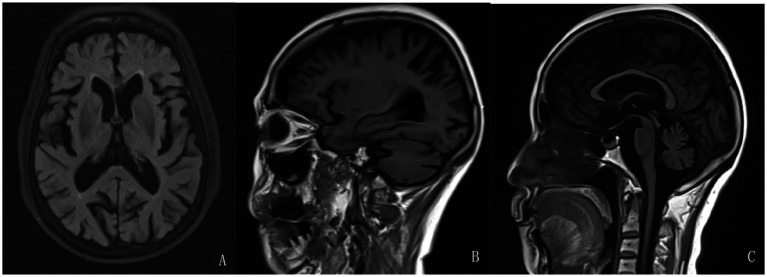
Brain magnetic resonance imaging (MRI) of the patient in 2022. Additionally, (A–C), have shown brain atrophy, ventricular enlargement, and widened, deepened, and enlarged cerebral sulci and fissures.
